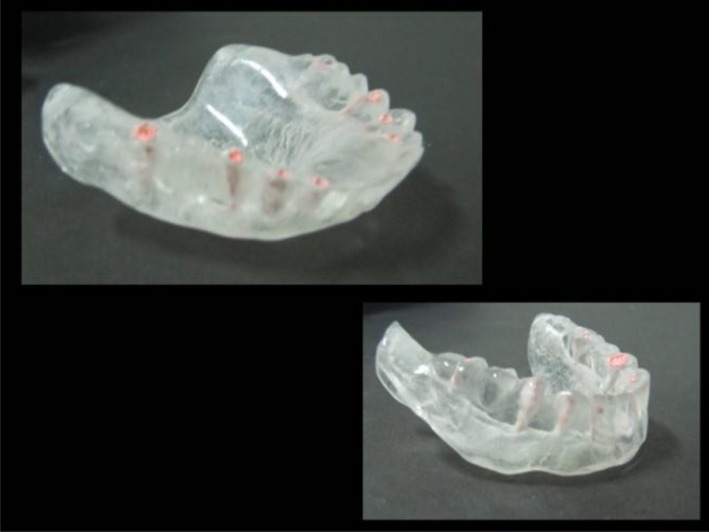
Radiographic stent for implant placement
